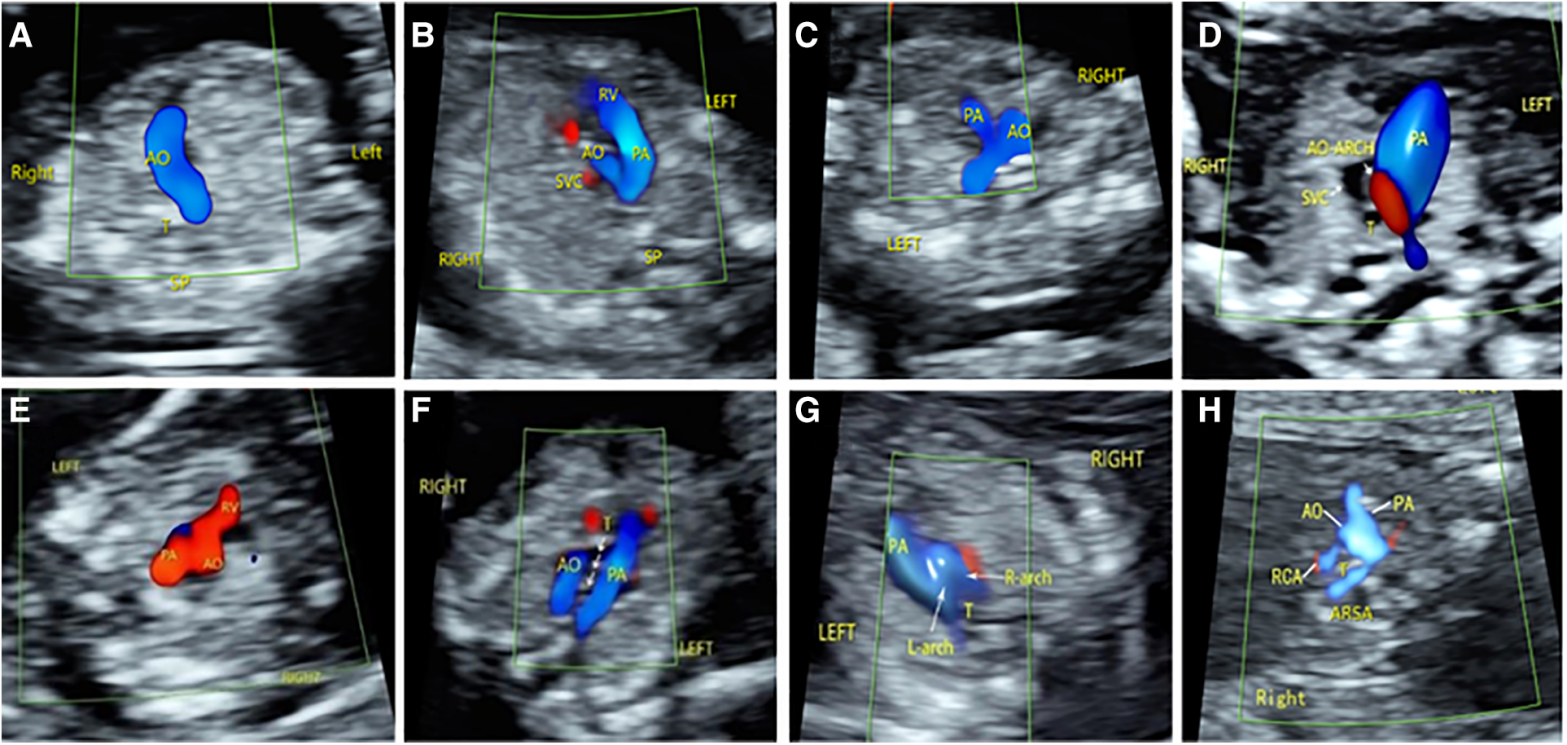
Abnormal ultrasound image pattern of 3VT section. (A) Patterns of 3VT-1, one vessel with a curved course or a straight course. (B) Patterns of 3VT-2: The aorta was smaller than the pulmonary artery. (C) Patterns of 3VT-3: The pulmonary artery was smaller than the aorta. (D) The aorta was smaller than the pulmonary artery, and reverse flow was observed in the aortic arch. (E) The “in–out sign” of the aorta and/or pulmonary artery. (F) Patterns of 3VT-6, two large arteries forming a “U” sign around the trachea. (G) Patterns of 3VT-7, two large arteries forming an “O” sign around the trachea. (G) Patterns of 3VT-8: The subclavian artery and aorta together form a “C” sign around the trachea. (H) Patterns of 3VT-8: The subclavian artery originates from the origin of the descending aorta and runs behind the trachea to form a “C”-type vascular ring.
